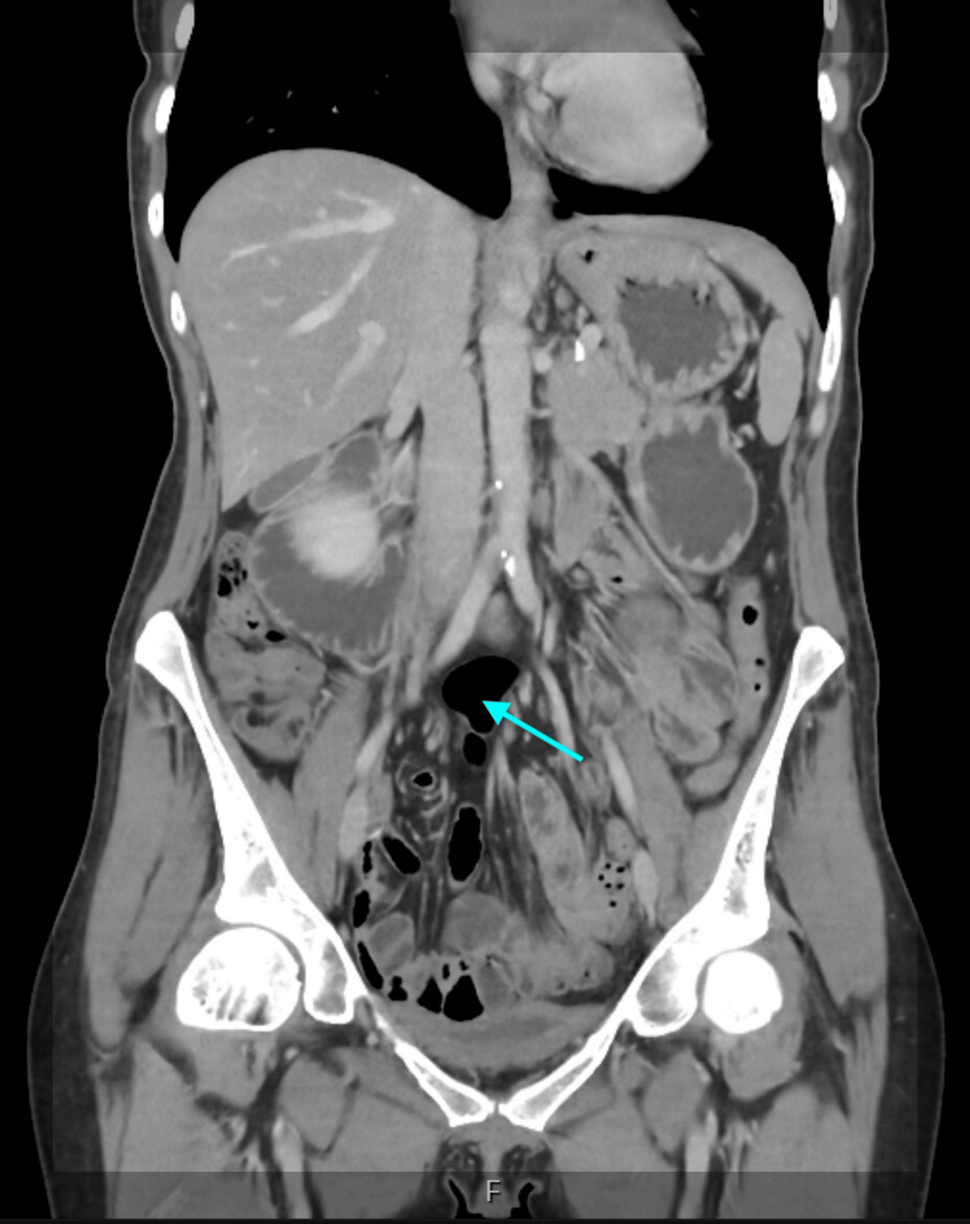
Coronal contrast-enhanced CT of the abdomen demonstrating a markedly distended stomach and proximal duodenum with abrupt narrowing of the third portion (arrow) as it traverses between the superior mesenteric artery and the aorta. This appearance reflects external vascular compression characteristic of superior mesenteric artery syndrome.
